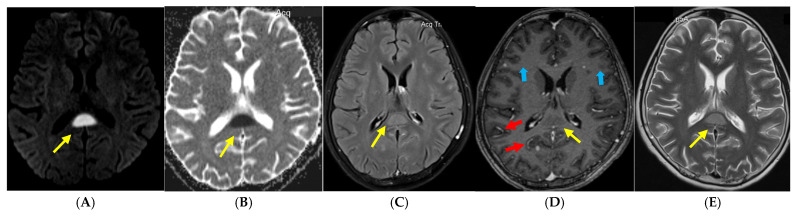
(A–E): Baseline MRI of a child with TB meningitis. Diffusion weighted (A), axial FLAIR (C) and Axial T2W (E) images showed a hyperintense, well-defined lesion in the splenium of the corpus callosum (yellow arrows). The same lesion was slightly hypointense on Axial T1W image and did not enhance with gadolinium (D). Corresponding lesion was hypointense on the apparent diffusion coefficient (ADC) map (B). Tuberculomas (blue arrows) and focal meningeal enhancement (red arrows) were also present.
